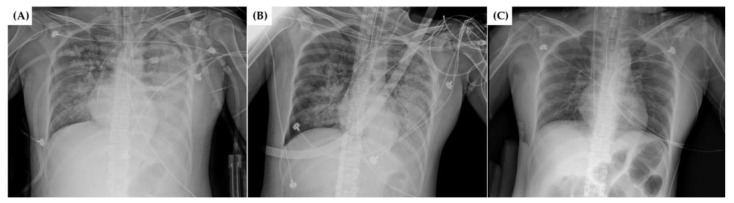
Chest radiography (A) before ECMO, (B) after ECMO, and (C) after ECMO weaned. ECMO: extracorporeal membrane oxygenation.
